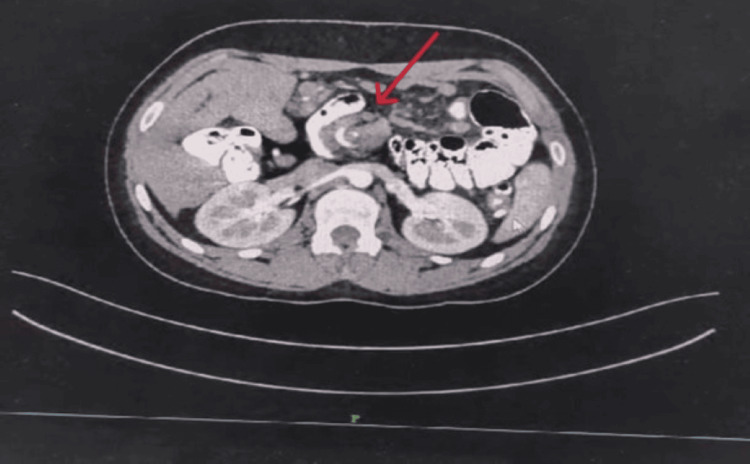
Whirlpool sign in the arterial phase of CECT CECT: contrast-enhanced computed tomography
